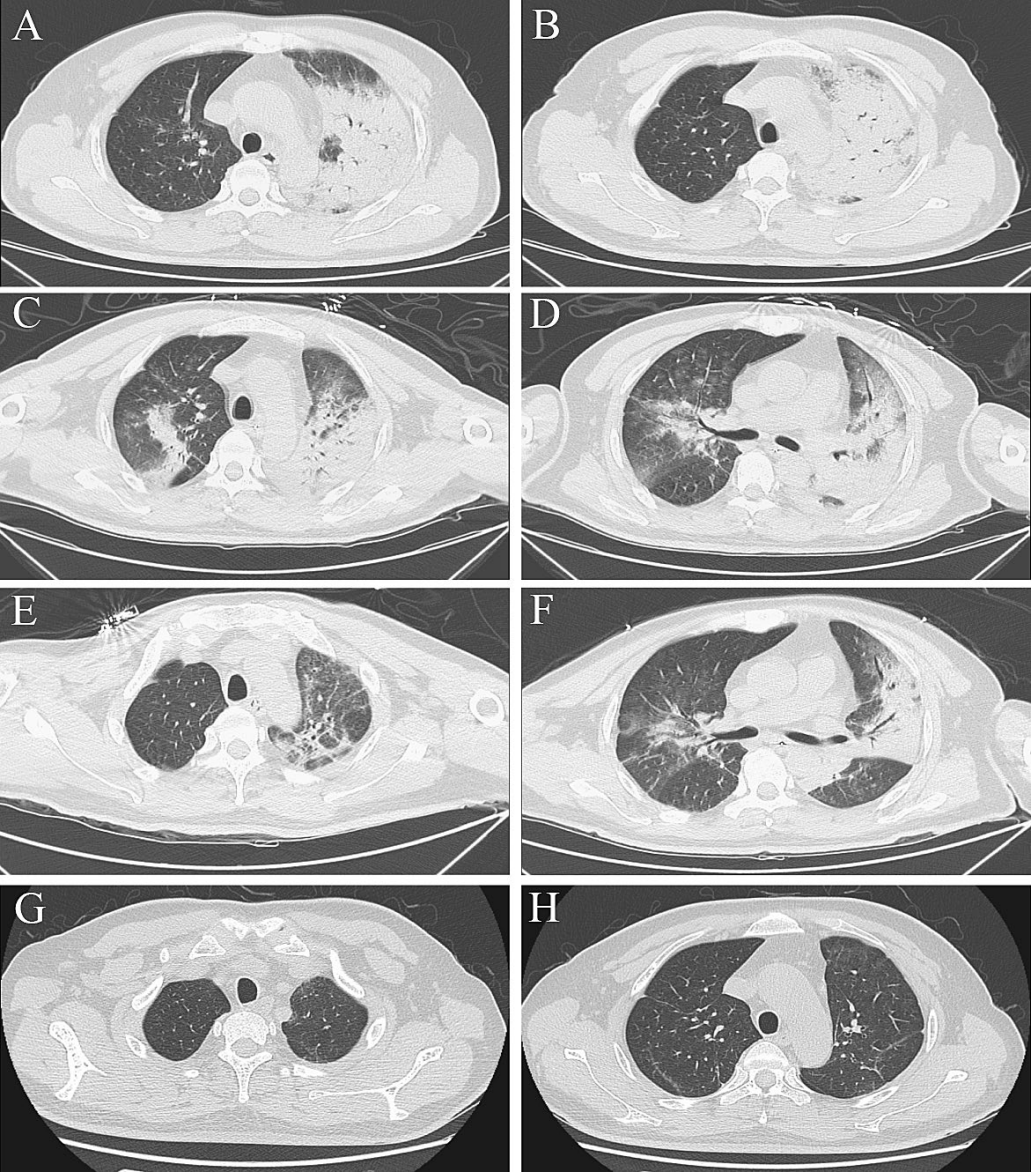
Serial chest computed tomography (CT) scans of a 43-year-old male farmer with severe psittacosis pneumonia. The initial CT scan (7 days after the onset) shows air-space consolidation with inflammatory exudation only appears in the superior lobe of left lung (a, b). The follow-up CT scan (16 days after the onset) shows exacerbation of the consolidated area in left lung and also in the middle and inferior lobes of right lung (c, d). On the follow-up CT scan (23 days after the onset), the area of consolidation has decreased (e, f) and 50 days after onset it has disappeared (g, h)
